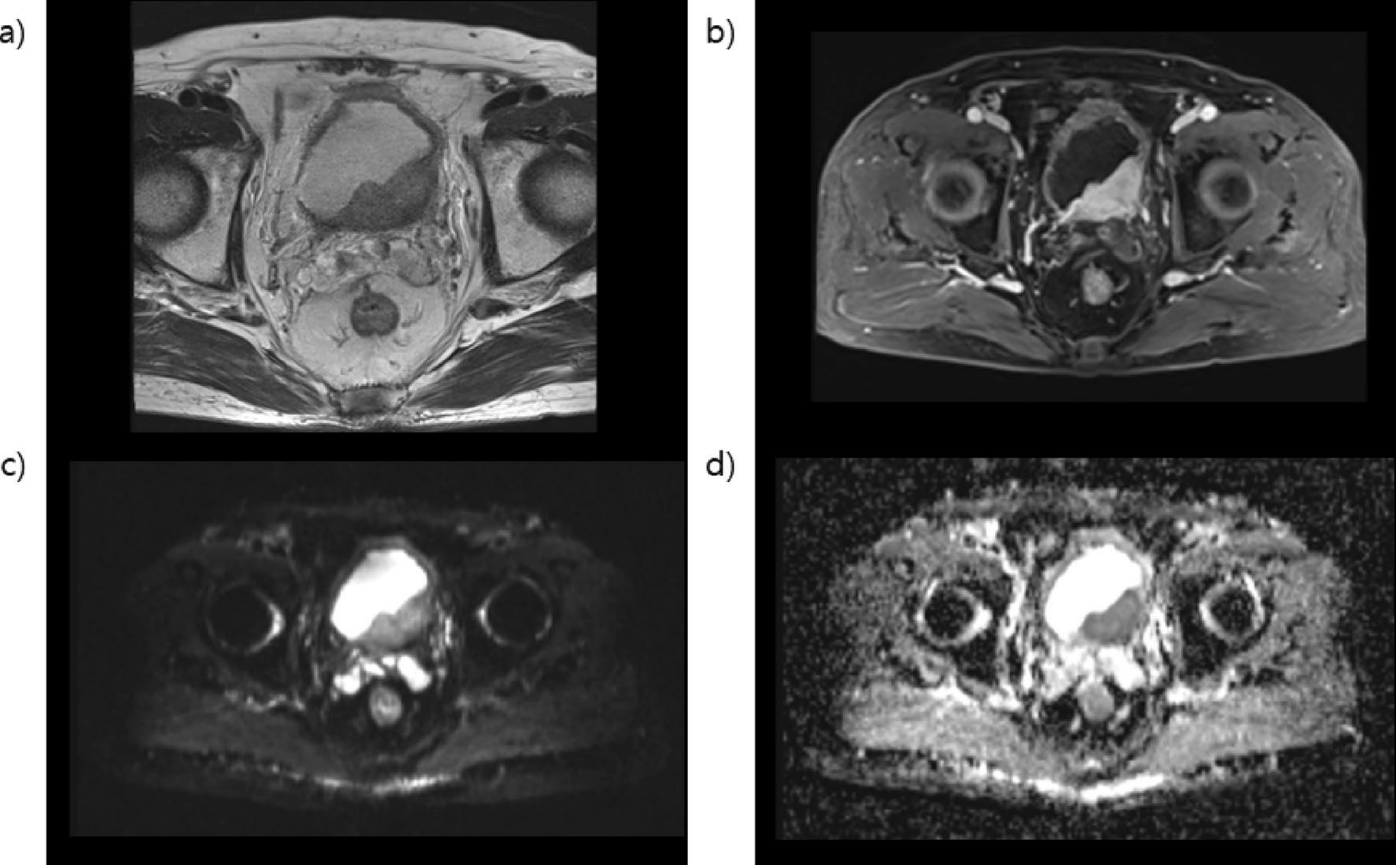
Magnetic resonance images of the extravesical tissue-invasive bladder cancer (a) T2-weighted image in the left posterolateral aspect of the bladder wall showing a bladder cancer invading underneath the hypointense detrusor lining to the extravesical fat tissue. (b) Dynamic contrast-enhanced image showing the enhancement of the lesion, invading the extravesical fat layer. (c) Diffusion-weighted imaging native image and (d) apparent diffusion coefficient map showing highly restricted diffusion of the mass with low signal intensity. There is an invasion of the entire bladder wall and extravesical fat. The multiparametric magnetic resonance imaging patterns are consistent with the extravesical tissue invasion of bladder cancer.
